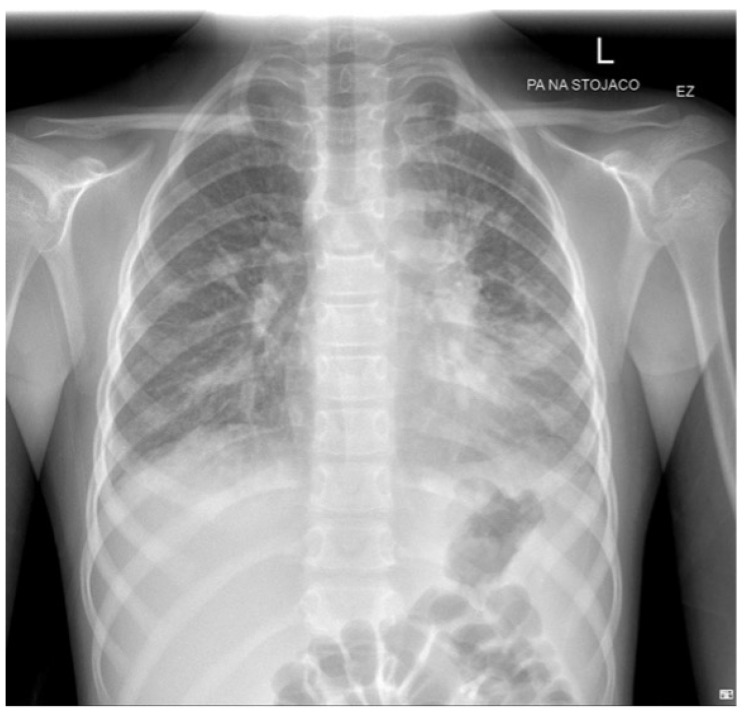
Chest X-ray: parenchymal and interstitial thickening within both lung fields spreading distally, most severe at the base of the left lung.
